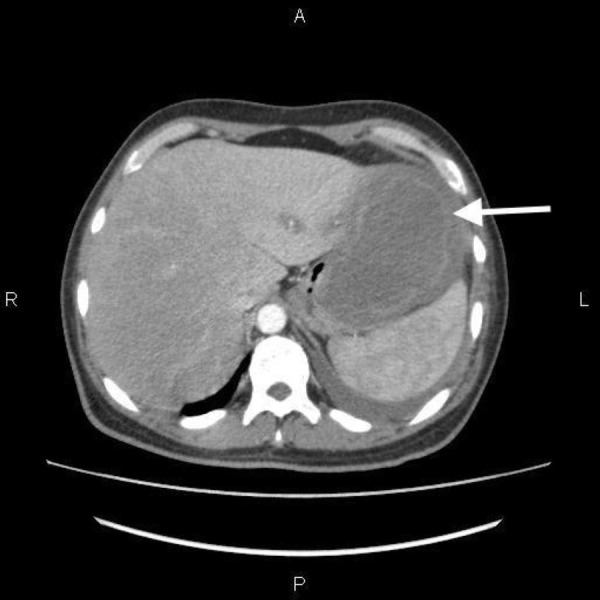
Computed tomography scan of the abdomen showing a large left-sided sub-phrenic collection involving the left lobe of the liver
